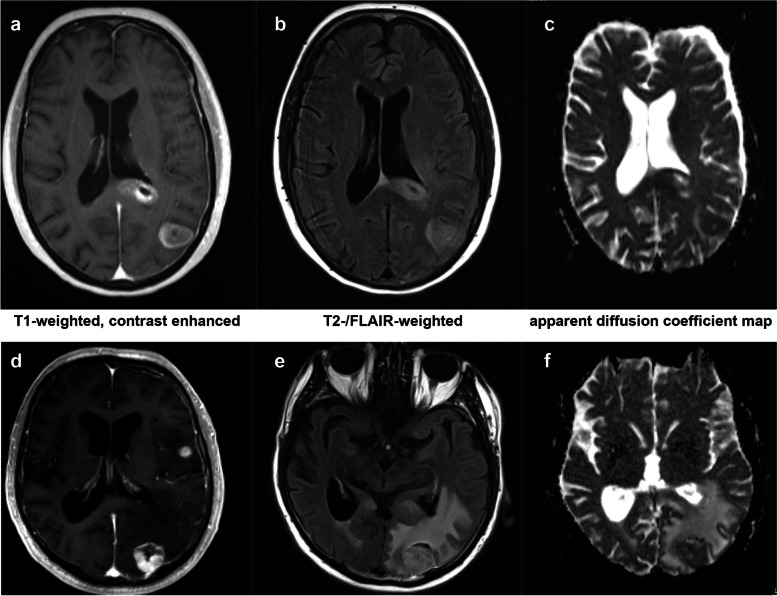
Example of two MRI of the tumor region and edema of a glioblastoma with multiple loci (a) – T1-weighted and contrast enhanced; (b) – T2/-FLAIR-weighted; (c) – ADC map) and of multiple brain metastases (d) – T1-weighted and contrast enhanced; (e) – T2/-FLAIR-weighted; (f) – ADC map). FLAIR – Fluid attenuated inversion recovery; ADC – apparent diffusion coefficient
